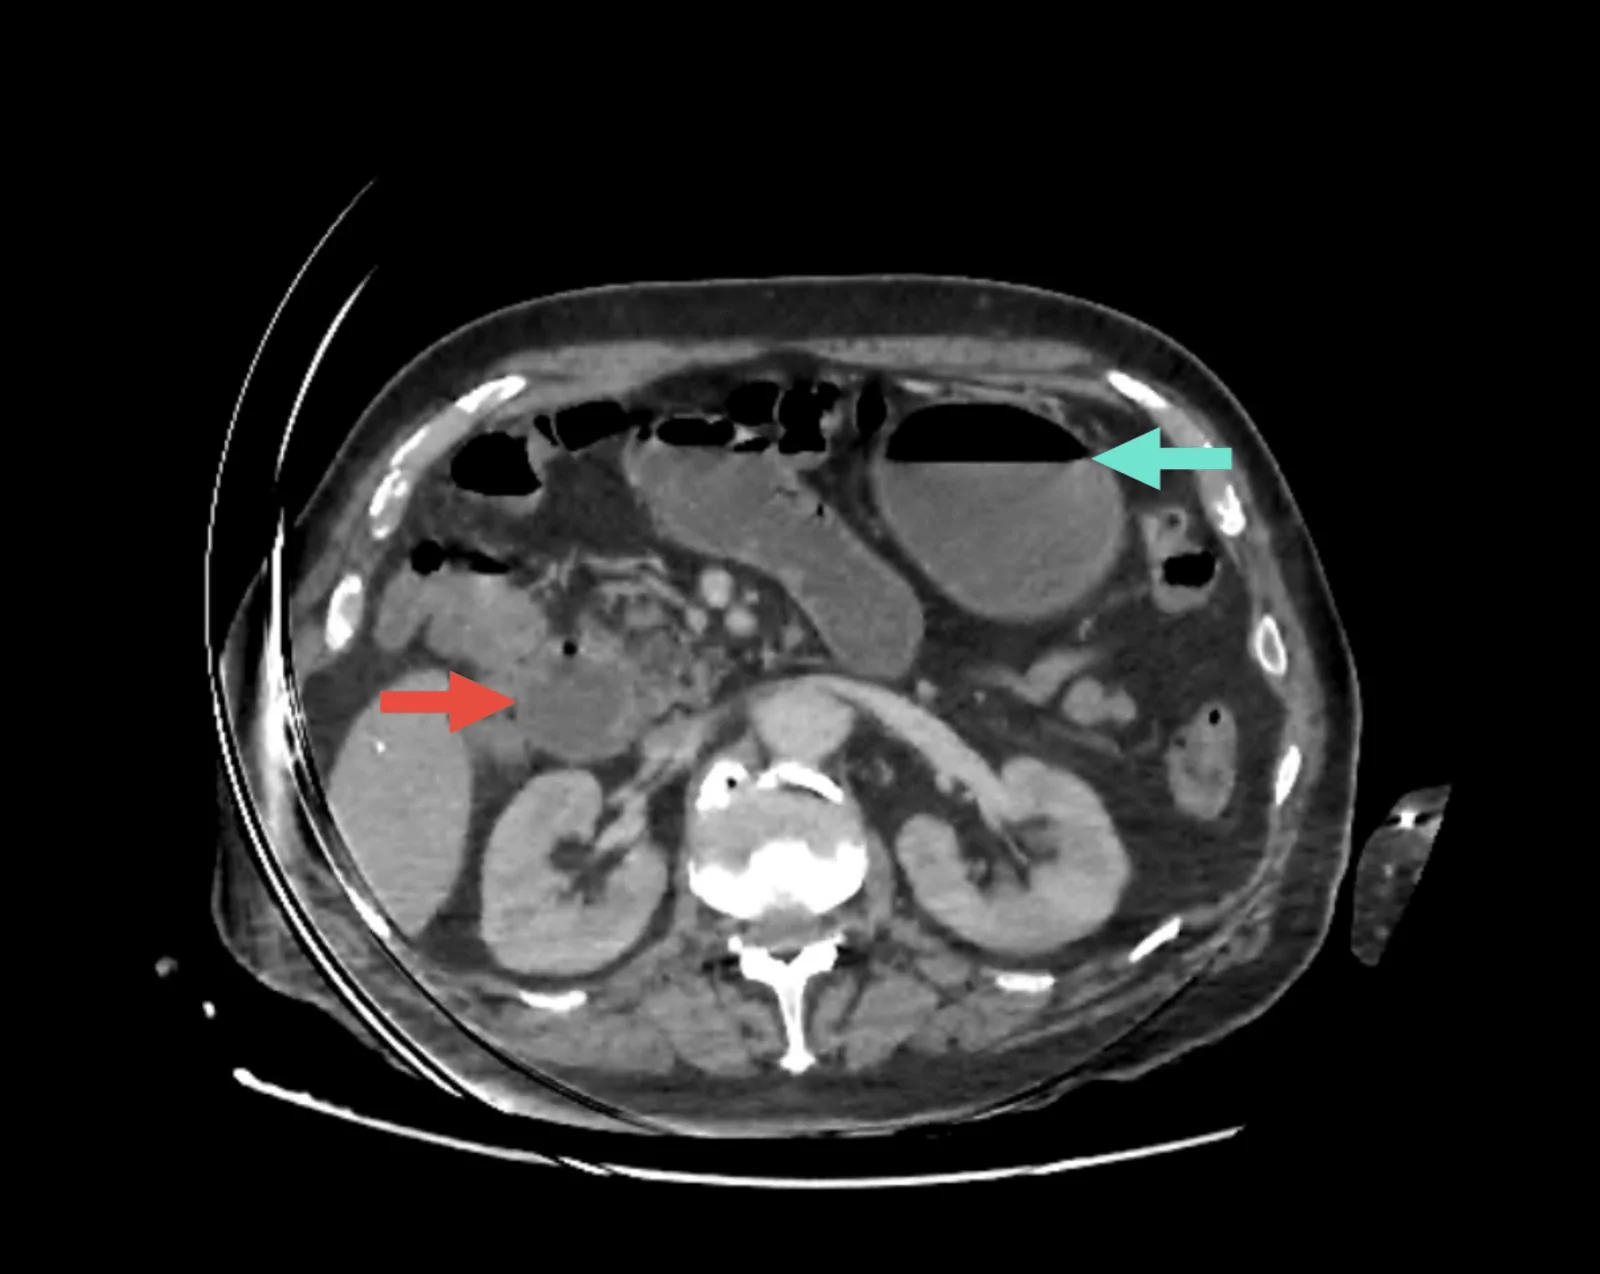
Axial non-contrast CT scan showing an intraluminal gallstone located in the jejunum associated with an air–fluid level. Red arrow: intraluminal gallstone; Blue arrow: air–fluid level
